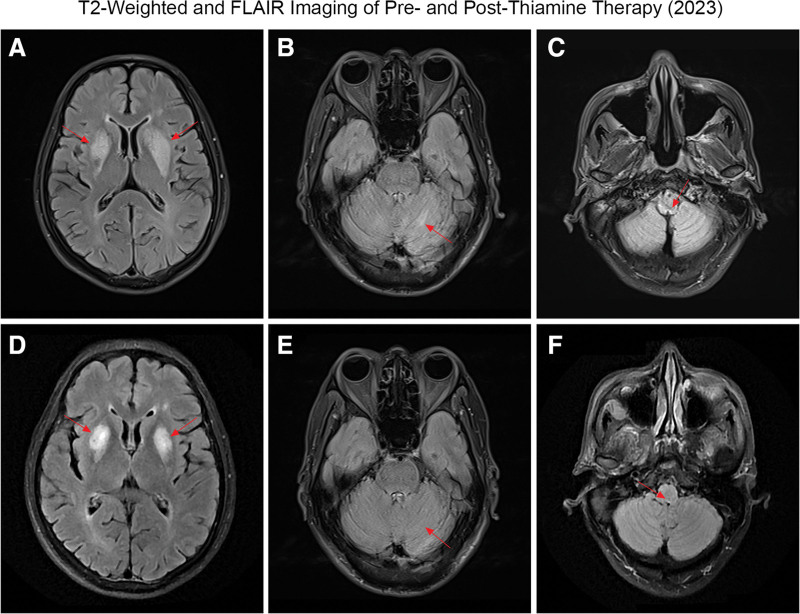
After thiamin cessation, MRI revealed that the bilateral basal ganglia lesions had progressed compared with the 1st hospitalization. Red arrows indicated hyperintense signals in bilateral basal ganglia lesions (A), the cerebellar dentate nucleus (B), and the dorsal medulla (C). After thiamin supplementation, hyperintense signals were decreased in bilateral basal ganglia lesions (D), the cerebellar dentate nucleus (E) and the dorsal medulla (F) on T2-weighted and FLAIR images. FLAIR = fluid-attenuated inversion recovery.
